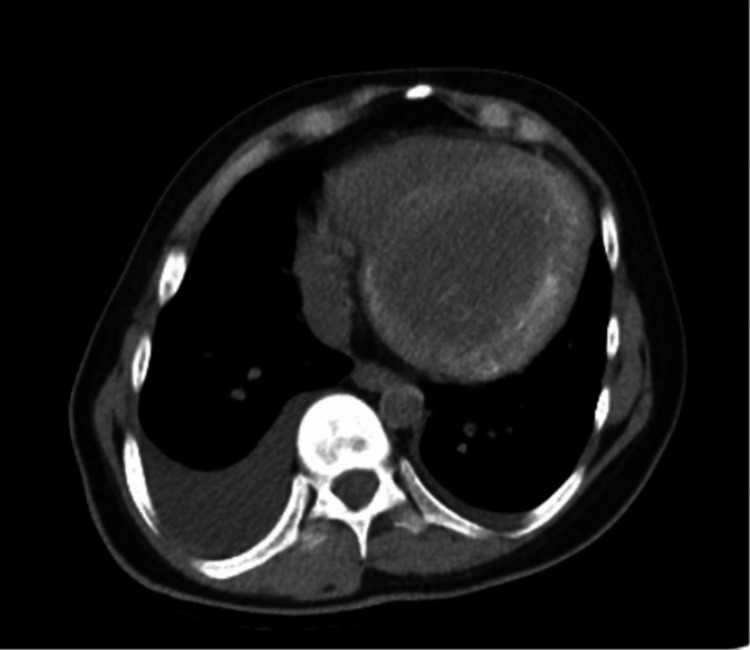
Non-contrast axial CT of the chest showing significant cardiomegaly with diffuse hyperdense calcifications in the left ventricular myocardium. Bilateral pleural effusions (more profound on the right) are also seen.
